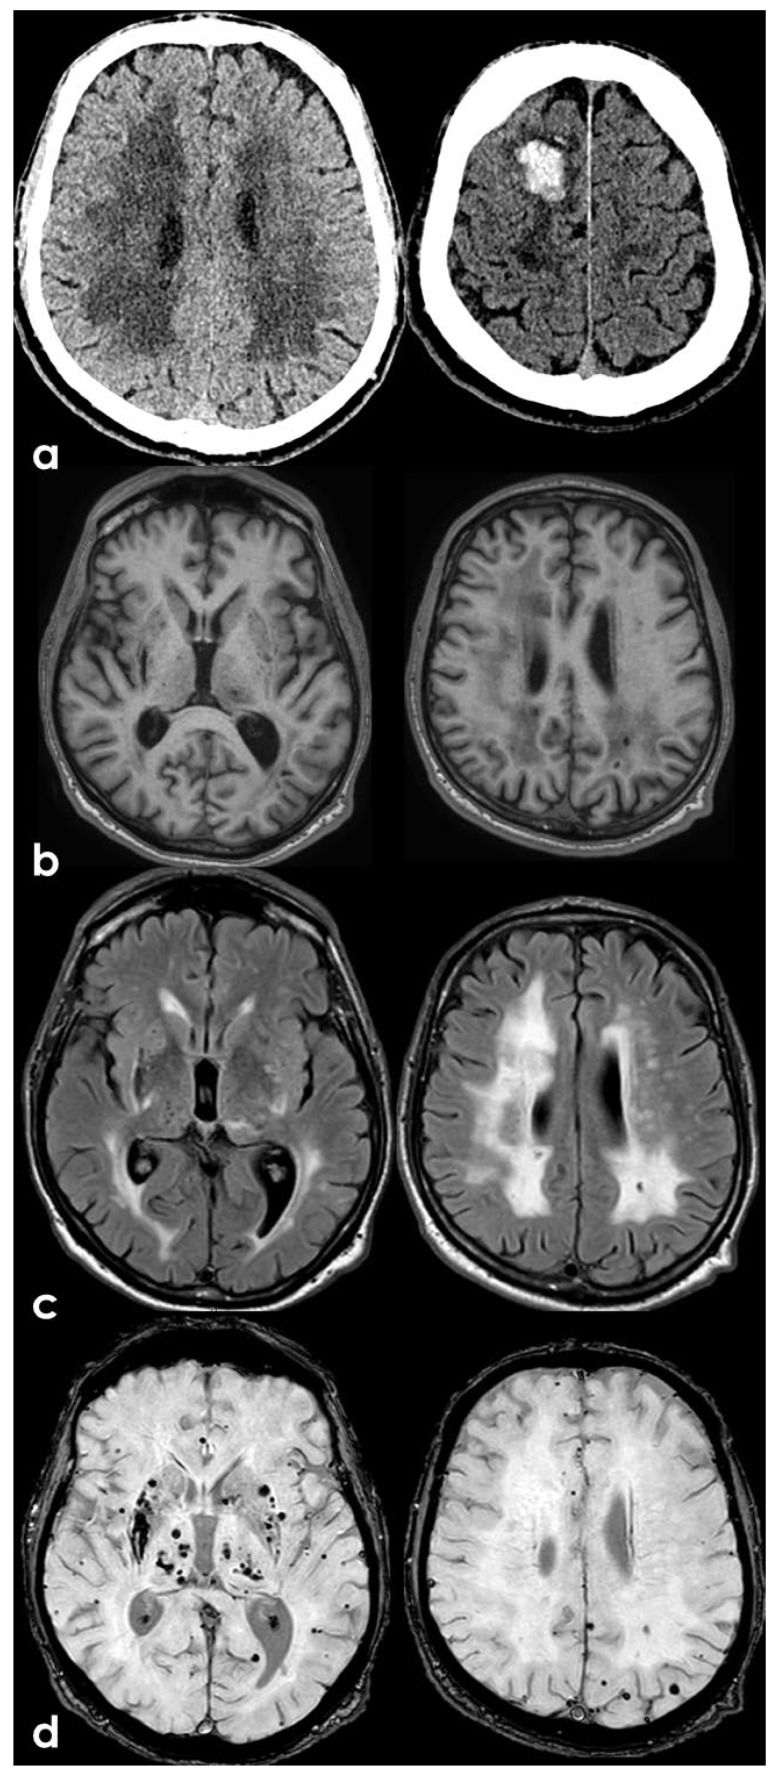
Example of CSVD associated with ABCC6 mutation in a 72-year-old woman without vascular risk factors (patient 15). Panel (a): non-contrast computed tomography (NCCT) of the brain showing extensive leukoaraiosis (grade 3 on the Fazekas scale) and a right frontal acute hemorrhage. Panels (b–d): brain magnetic resonance imaging (MRI) axial slices at the same two levels using different sequences [(b) T1W; (c) T2-fluid-attenuated inversion recovery—FLAIR; (d) susceptibility weighted imaging (SWI)], showing lacunes (b,c), leukoaraiosis (c), and several deep and lobar microbleeds (d).
